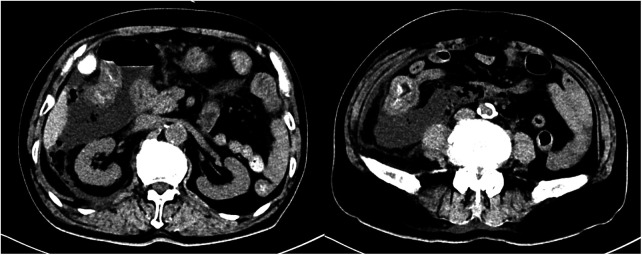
Abdominal CT scan showing retroperitoneal gas and fluid accumulation.
